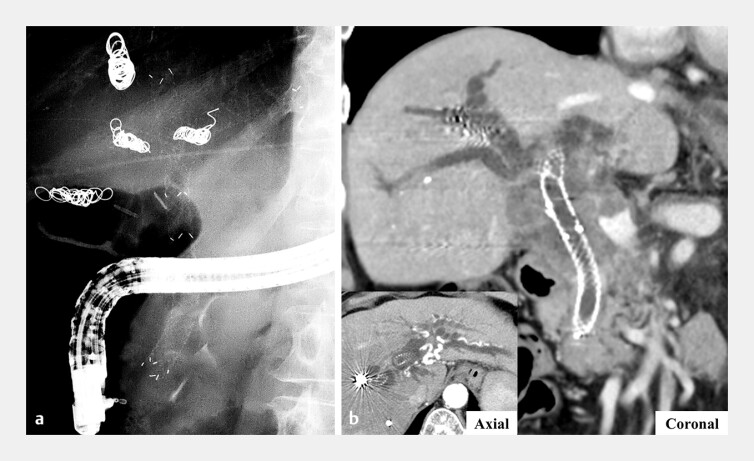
Fluoroscopic and computed tomographic (CT) images.
